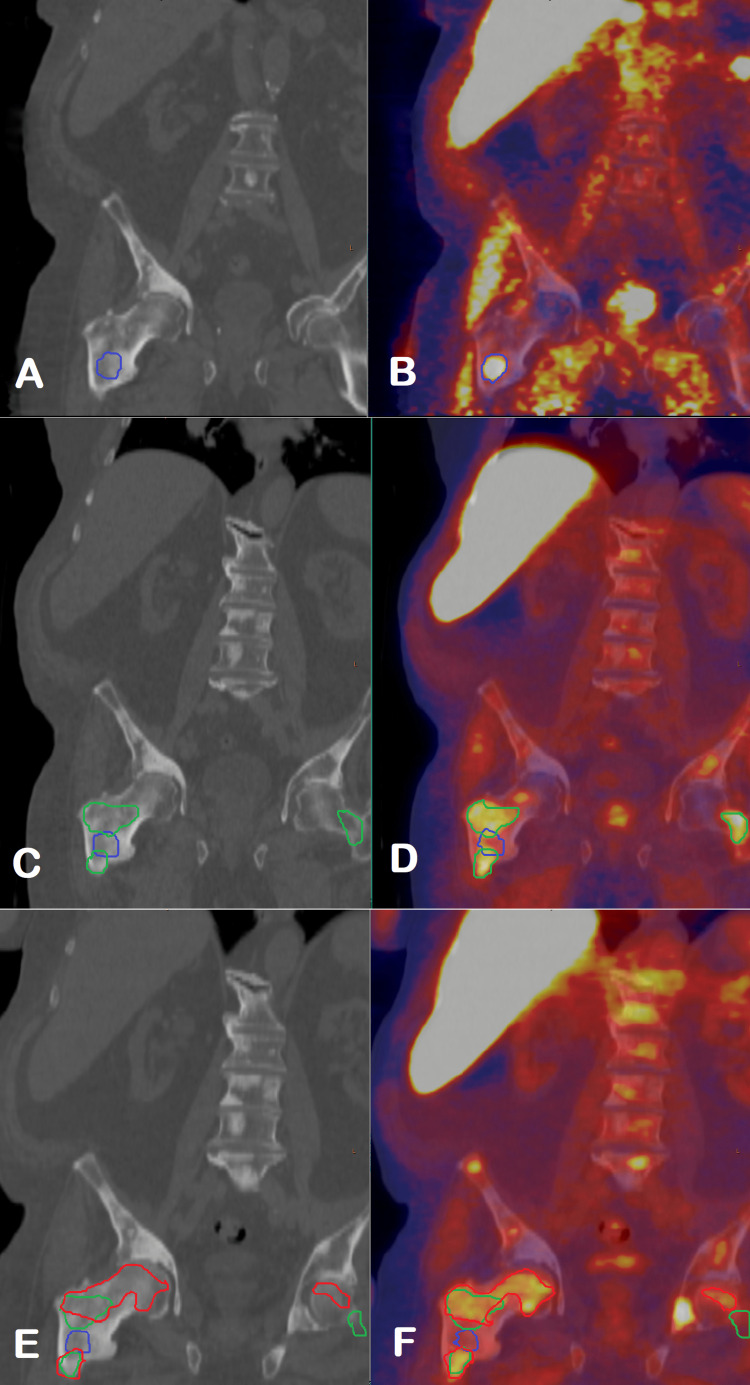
Changes in osseous lesions on Fluoride-18 fluciclovine PET-CT scan after treatment with sipuleucel-T CT (A) and fused PET-CT (B) images from October 2021 prior to sipuleucel-T treatment show an intense focal radiotracer activity in the intertrochanteric region of the right femur, corresponding to a new faintly dense lesion (blue outline), adjacent to a more densely sclerotic non-active lesion, which was present on imaging from October 2020. In the first follow-up study after sipuleucel-T treatment in April 2021, CT (C) and fused PET-CT (D) images show increased extent of sclerosis and associated abnormal radiotracer activity in the intertrochanteric region of the right femur (green outline), although activity in the previously active lesion decreased (blue outline). There is also a new area of faint sclerosis in the left femoral neck with intense radiotracer activity. On the CT (E) and fused PET-CT (F) images from June 2022, there is complete resolution of previously seen abnormal activity in the initially active lesion in the right hip (blue outline), decreased intensity of activity in the adjacent sclerotic area, and in the left femoral neck region (green outline). However, new areas of sclerosis and metabolic activity are seen (red outline).
